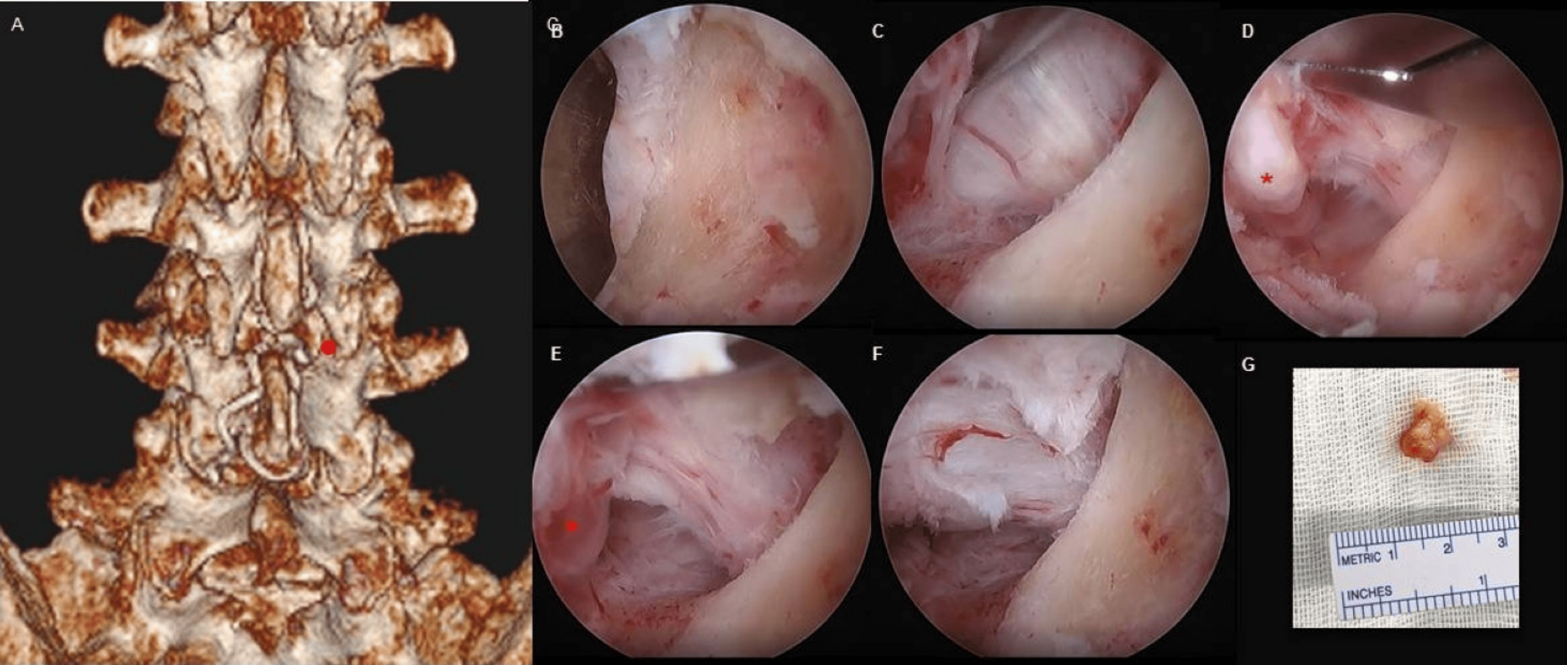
UBE Target Location at the Lateral Recess Junction Zone CT reconstruction of the lumbar spine of a patient with a previous ILP placement. The red dot indicates the location corresponding to the right lateral recess (A). Intraoperative images L3-4 level and endoscopic technique sequence for the FC identification and resection;  initial target area location (B), clear cleavage plane preparation between the lesion and the dura (C), FC (red asterisk *) with typical outpouch appearance arising from the joint capsule with surrounding adhesions (D), traversing nerve root widely exposed with surrounding structures visualized (red dot) (E), final decompression state (F), and macroscopic appearance of the resected FC (G). UBE: unilateral biportal endoscopy, ILP: interspinous ligamentoplasty, FC: facet cyst.
